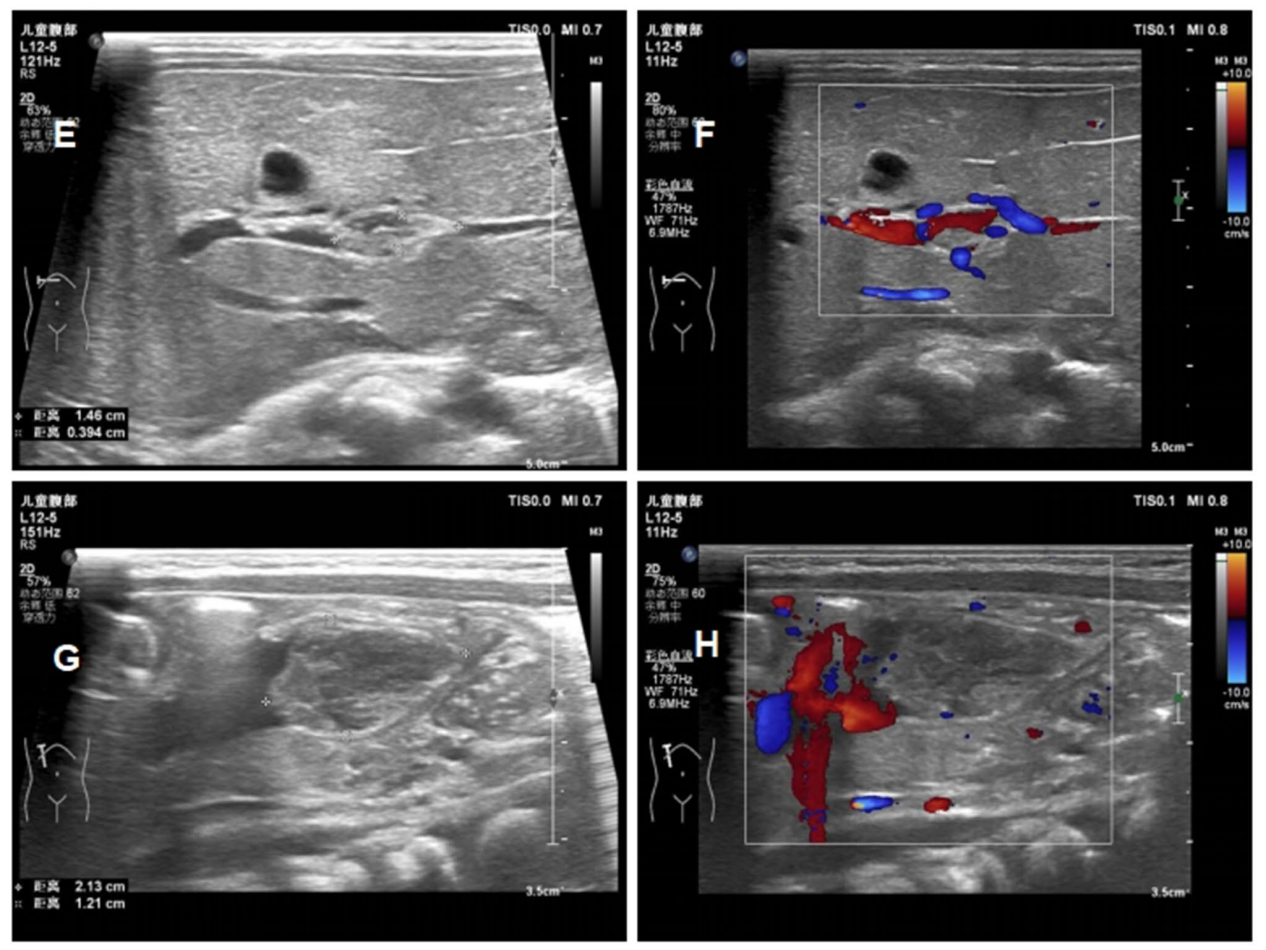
Ultrasound images of the patients ont day 16 of life (E) reduction in the size of the portal vein thrombus at day 16; (F) the blood flow signal of portal vein thrombosis at day 16; (G) reduction in the size of the umbilical vein thrombus at day 16; (H) the blood flow signal of umbilical vein thrombosis at day 16.
